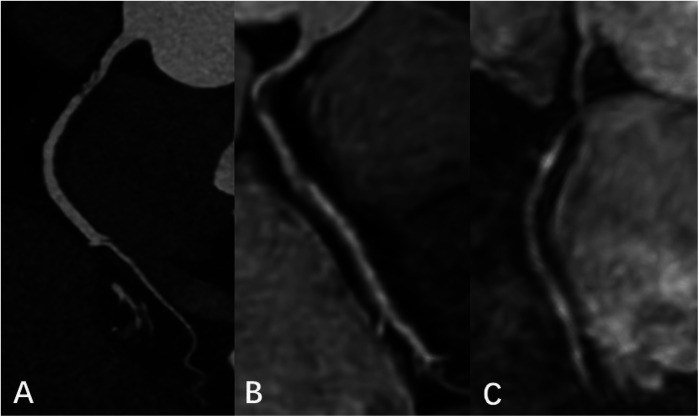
Male, 66 Y, with moderate stenosis of RCA. CTA (A) showed mixed plaque in the proximal segment of RCA with moderate lumen stenosis. 3D BTFE (B) and MSG-EPI (C) also showed local moderate stenosis of the RCA proximal segment.
